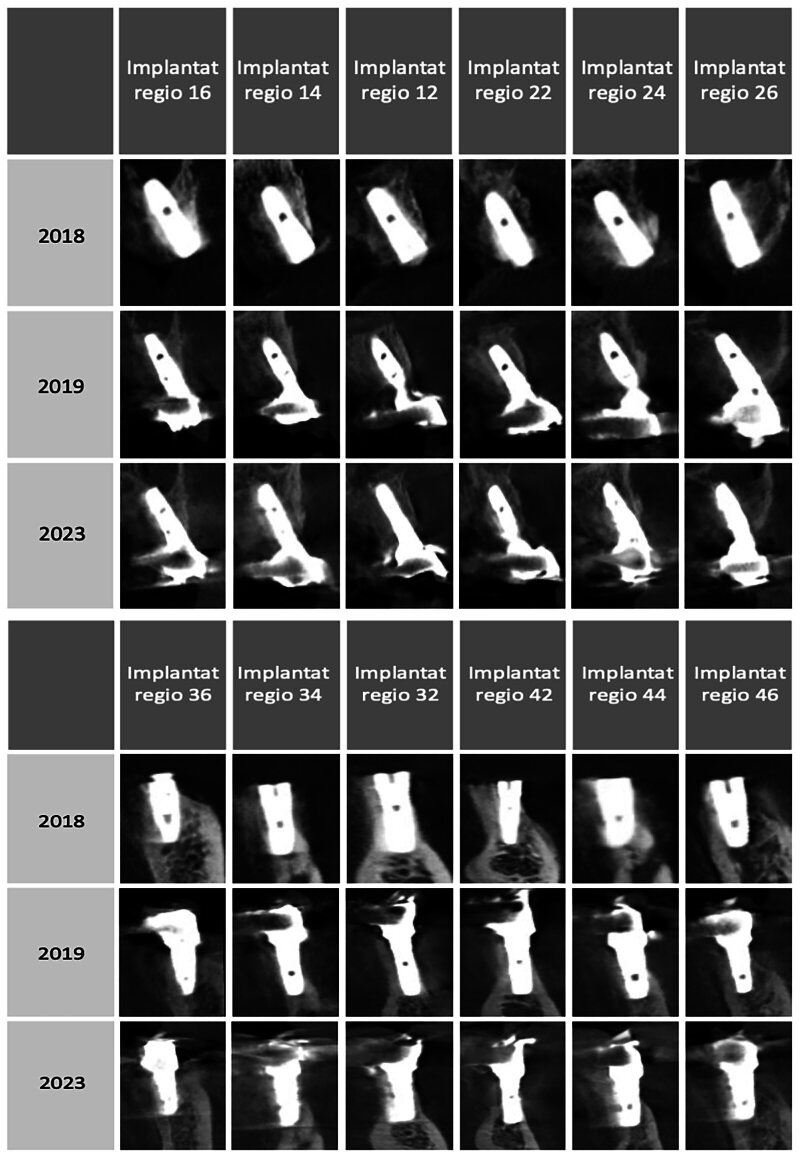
CBCT follow-up of individual implants.
